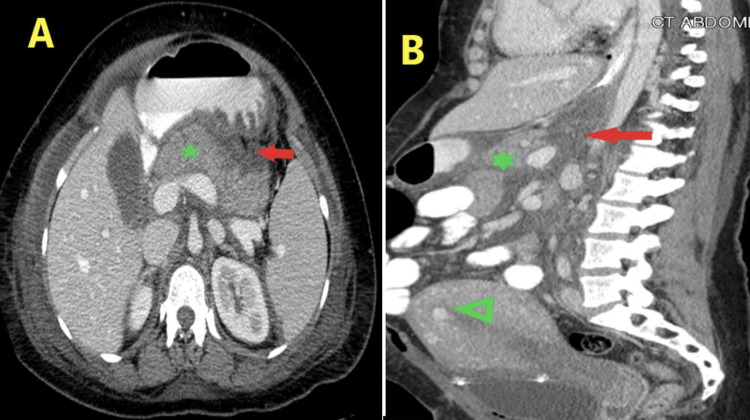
CT of the abdomen with contrast. A: Diffusely edematous and swollen pancreas (green star) with loss of its feathery appearance and associated smudged surrounding fat planes and mild prominence of the CBD. B: Partially encysted peripancreatic and lesser sac fluid collection (red arrow) and postdelivery bulky uterus (green arrowhead). CT: computed tomography, CBD: common bile duct
